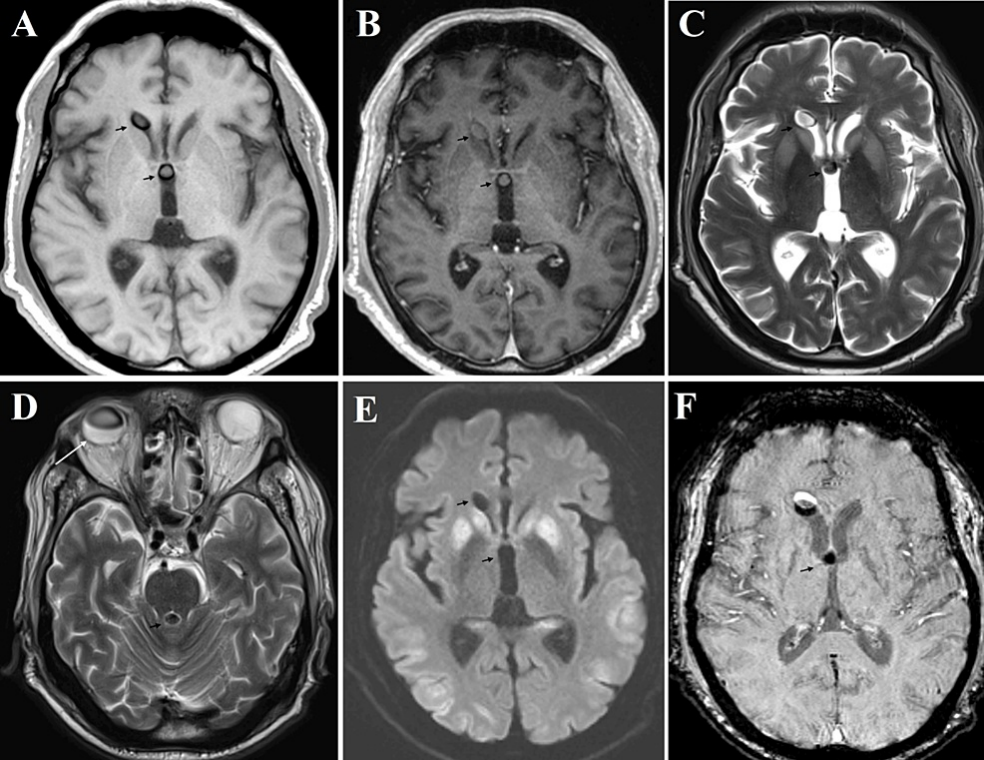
Axial slices of magnetic resonance imaging with black arrows delineating intraventricular lesions, most consistent with migrated vitreous silicone oil T1 precontrast imaging demonstrates isointense to grey matter intraventricular lesions (A) which do not contrast enhance on T1 postcontrast imaging (B). These lesions are iso- to hypointense to grey matter on T2 imaging (C, D) and do not diffusion-restrict on diffusion weight imaging (E) There is susceptibility artifact on susceptibility weighted imaging (F). Changes of a right vitrectomy are evident on T2 imaging (D)
